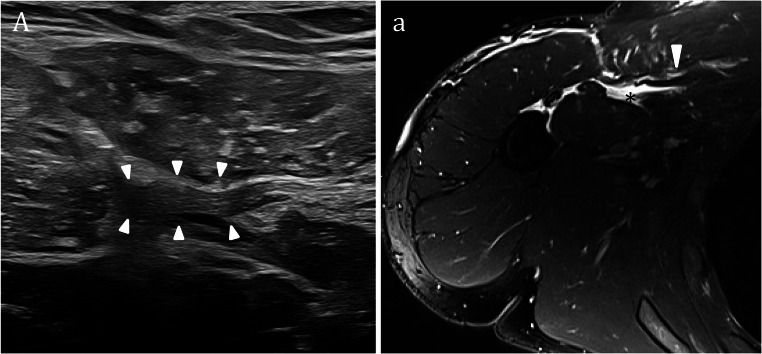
Complete tear of pectoralis major tendon. A-a Axial US image and axial fat saturation PD MRI images demonstrate a full-thickness tear of the pectoralis major tendon (arrowhead) with complete retraction from the humeral insertion and associated fluid collection (*)
